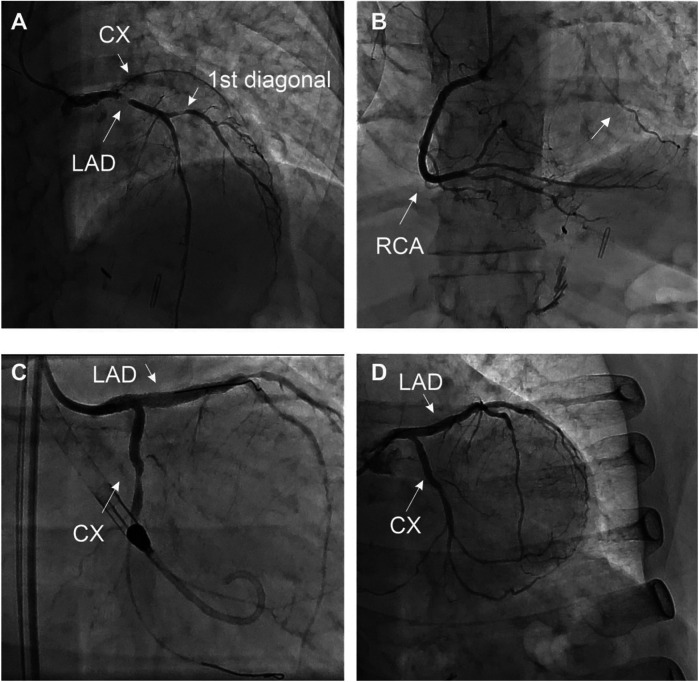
Coronary angiography at the initial presentation (A–C) and final result (D) the patient presented with extended 2-vessel coronary artery disease with a 50%–70% lesion of the distal LM, a sub-occlusive lesion of the proximal LAD with diffuse atherosclerotic infiltration of the median and distal part, à 70% stenosis of the 1st diagonal, and a CTO of the LCX collateralized by the RCA (arrow). MT, LAD, and LCX were initially treated by PCI under mechanical circulatory support (Impella CP system®). The 1st diagonal was treated during the second angiography (D), after documentation of the residual ischemia by PET-CT (Figure 4).
